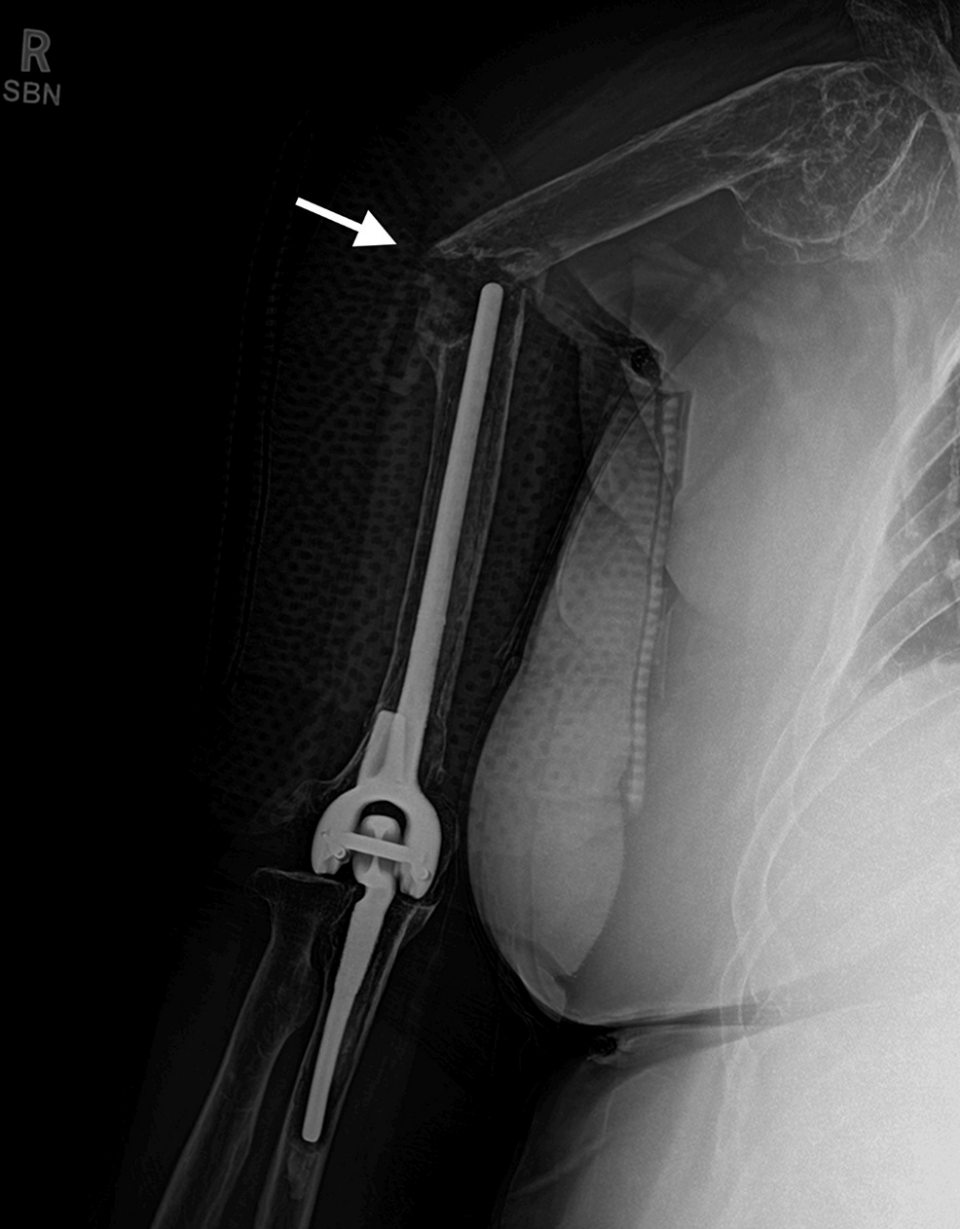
Preoperative radiograph of periprosthetic humerus fracture. Arrow designates periprosthetic humerus fracture.
